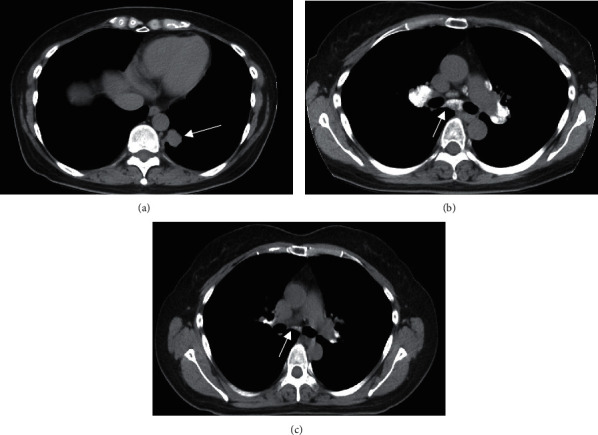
CT scan showing the primary lung tumor (a), calcification of the mediastinal lymph nodes before the administration of alectinib (b), and the lymph nodes 4 months after the administration of alectinib (c). White arrow showing subcarinal lymph nodes (b, c). CT: computed tomography.
